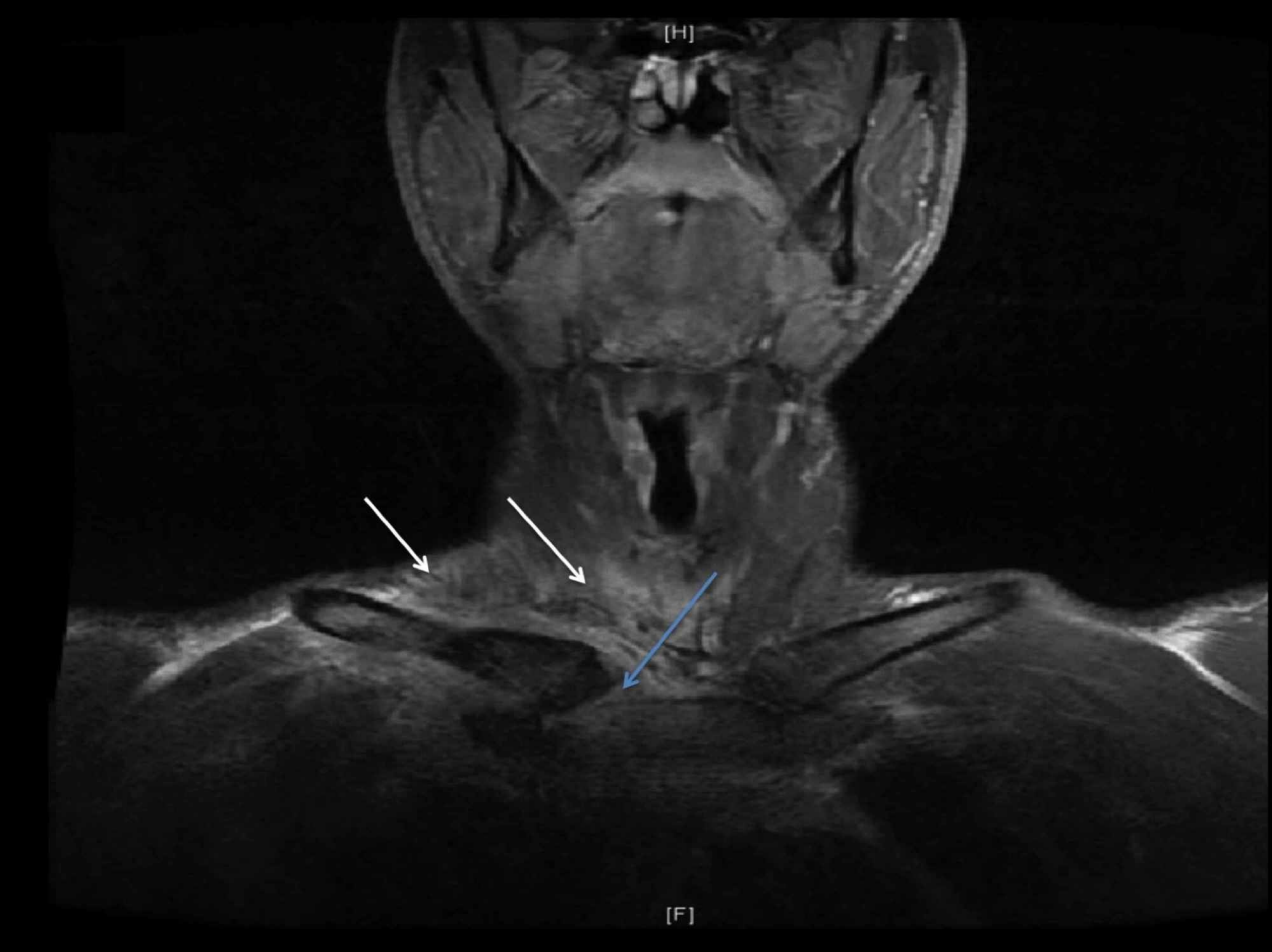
MRI of the neck and SCJ with contrast. MRI of the neck and SCJ with contrast showed large infiltrative process involving the right SCJ (blue arrow) and the surrounding soft tissues with extension into the base of the neck on the right side of the midline (white arrows). SCJ, sternoclavicular joint
